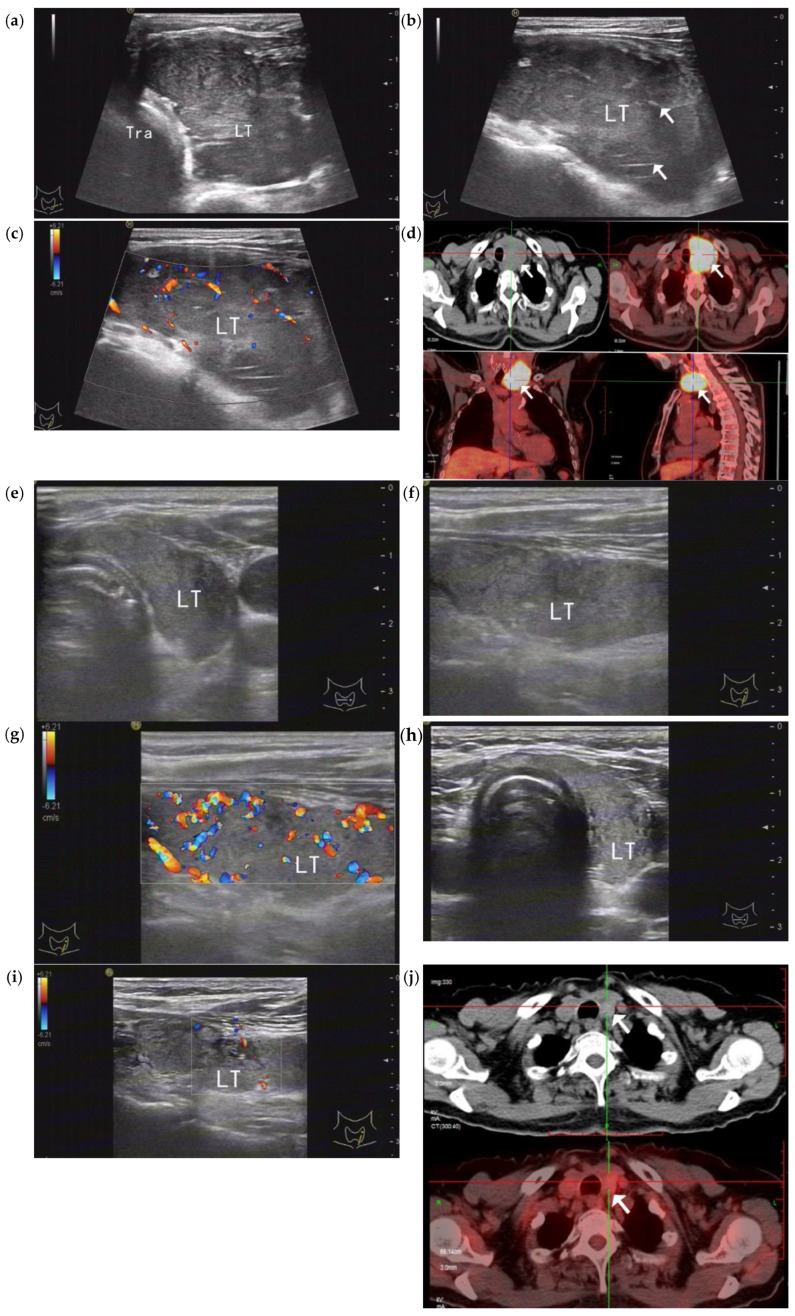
An elderly woman with diffuse--type PTL (DLBCL subtype) in the LT who underwent a right thyroidectomy 17 years ago due to Hashimoto’s thyroiditis. Regular follow-ups with ultrasound indicated a significant enlargement of the LT. (a,b) Gray-scale ultrasound of the transverse and longitudinal sections before chemotherapy shows a markedly increased LT volume, with a maximum thickness of 3.6 cm and internal “stripe-shaped” high echoes (arrows). (c) CDU showing abundant blood flow signals. (d) PET-CT before chemotherapy showing significant enlargement of the LT and an abnormal radiation uptake increase (arrows), with an SUVmax of 25.9. (e–g) After two cycles of the R-CHOP regimen, the LT thickness significantly decreased to 1.7 cm, and the parenchymal echo increased but still showed rich blood flow signals. Considering the treatment’s effectiveness, continuing the original chemotherapy regimen was recommended. (h,i) After four cycles of chemotherapy, the ultrasound showed a normal thyroid thickness, an increased glandular echo, which had returned to normal, the disappearance of the lesion, and a decreased blood flow signal. (j) PET-CT confirmed no metabolic increase in the lesion, with an SUVmax of 2.0 (arrows). PTL, primary thyroid lymphoma; DLBCL, diffuse large B-cell lymphoma; LT, left lobe; CDU, color Doppler ultrasound; PET-CT, positron emission tomography-computed tomography; SUVmax, maximum standardized uptake value; R-CHOP, rituximab, cyclophosphamide, doxorubicin, vincristine, and prednisone.
